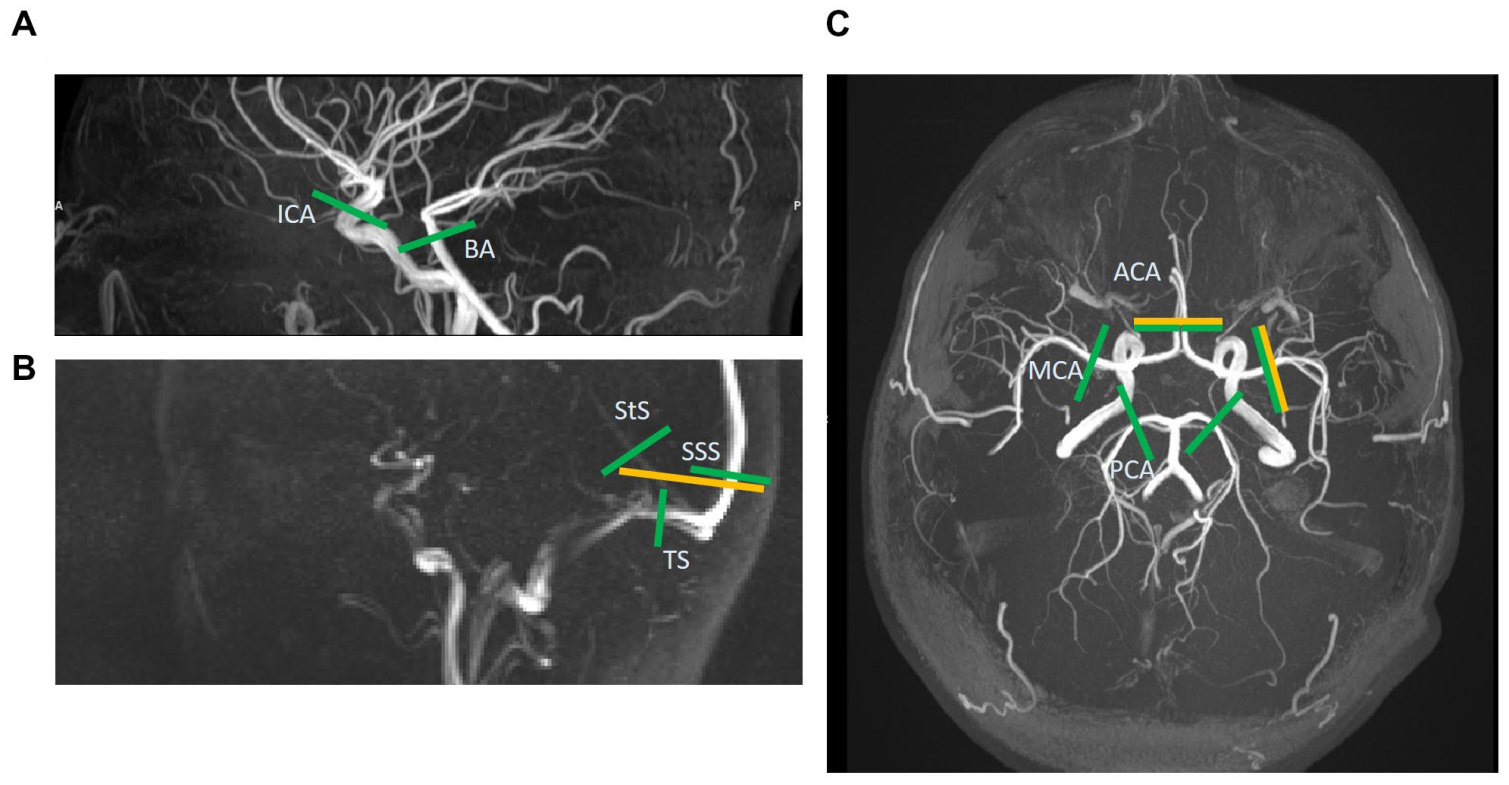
Slice locations of 2D phase-contrast MR images (yellow) and those extracted post-hoc from the 4D flow volume (green). A total of five vessels are assessed with 2D and 13 with 4D. (A) This sagittal time-of-flight (TOF) image shows the internal carotid and basilar artery slices (ICA & BA). (B) This sagittal low-res vessel scout image shows the venous sinuses more clearly than the TOF. Here the 2D slice across the superior sagittal and straight sinuses (SSS & StS) can be seen. These two vessels, as well as the slices across the transverse sinuses (TS), are shown for the 4D image too. (C) This axial TOF image shows the 2D slices for the right middle cerebral artery (MCA) and combined anterior cerebral artery (ACA) images, as well as the 4D slices for the ACAs, MCAs, and posterior cerebral arteries (PCA).
